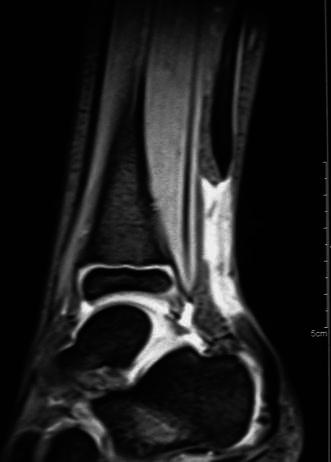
A chronic full-thickness tear of the right Achilles tendon with a gap of 5 cm was present on MRI
